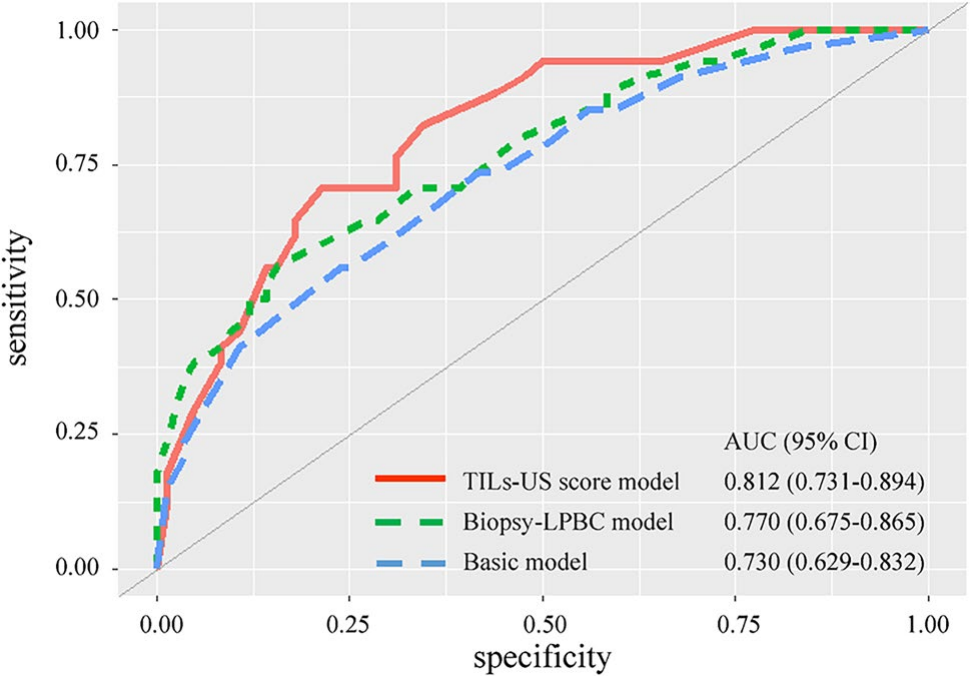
Receiver operation curves of prediction nomograms for a pathological complete response. The areas under curves are compared with the Delong test. *AUC* area under the curve, *LPBC* lymphocyte-predominant breast cancer, *TILs–US* tumor-infiltrating lymphocytes–ultrasonography
